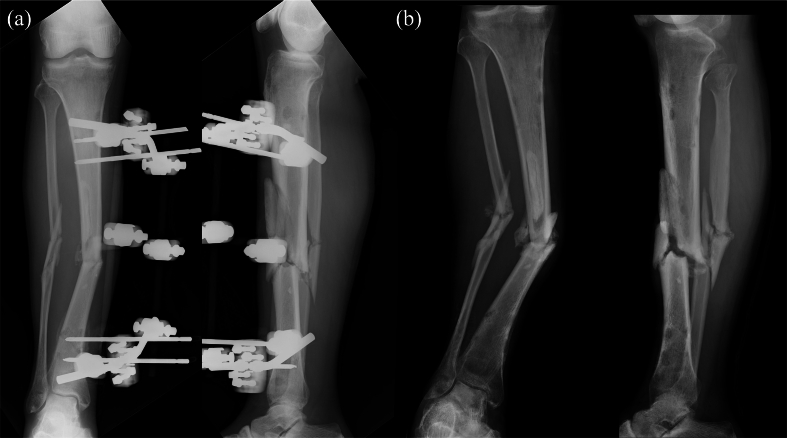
Radiographic images (a) immediately following the application of the external fixator and (b) at the time of the first visit to our department.
